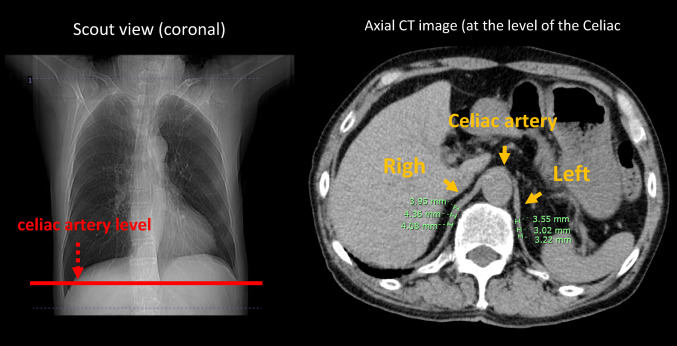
Representative CT image used for the measurement of diaphragmatic crus thickness. Diaphragmatic crus thickness was measured bilaterally at the level of the celiac artery on axial CT images. Measurements were obtained at the anterior, middle, and posterior portions of each crus, and the mean value of six measurements was used for analysis. CT, computed tomography
